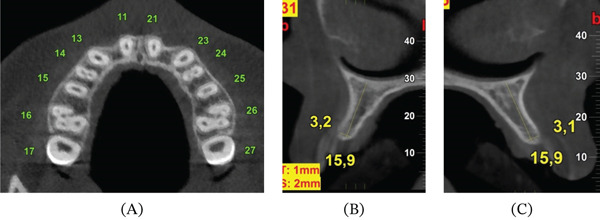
(A–C) CBCT images at the beginning of the retention period.
